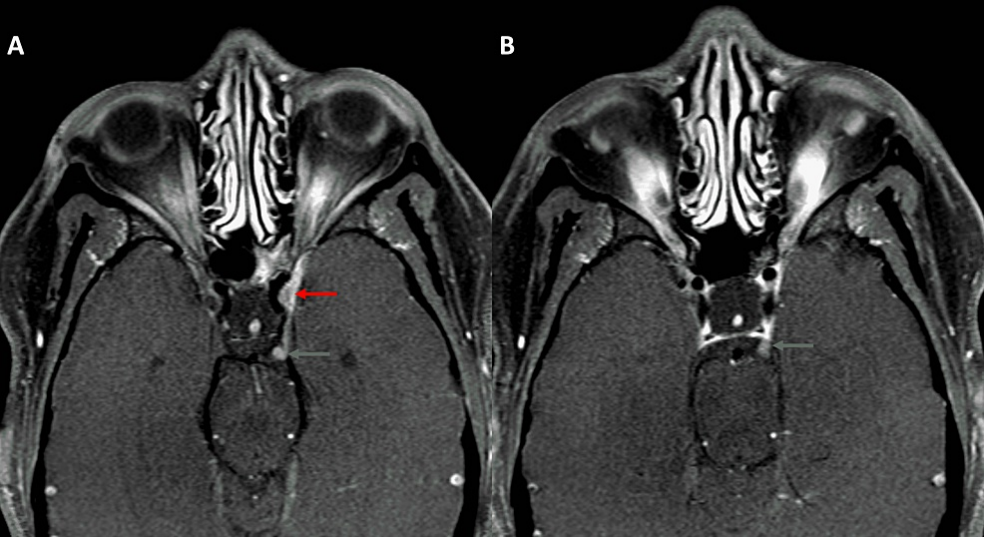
Postcontrast axial T1-weighted images (T1WI) showing nodular thickening in the region of the cavernous (red arrow in A) and cisternal segment (green arrows in A and B) of the left oculomotor nerve Note that nodular thickening of the cisternal segment was visible on two consecutive images (both A and B).
